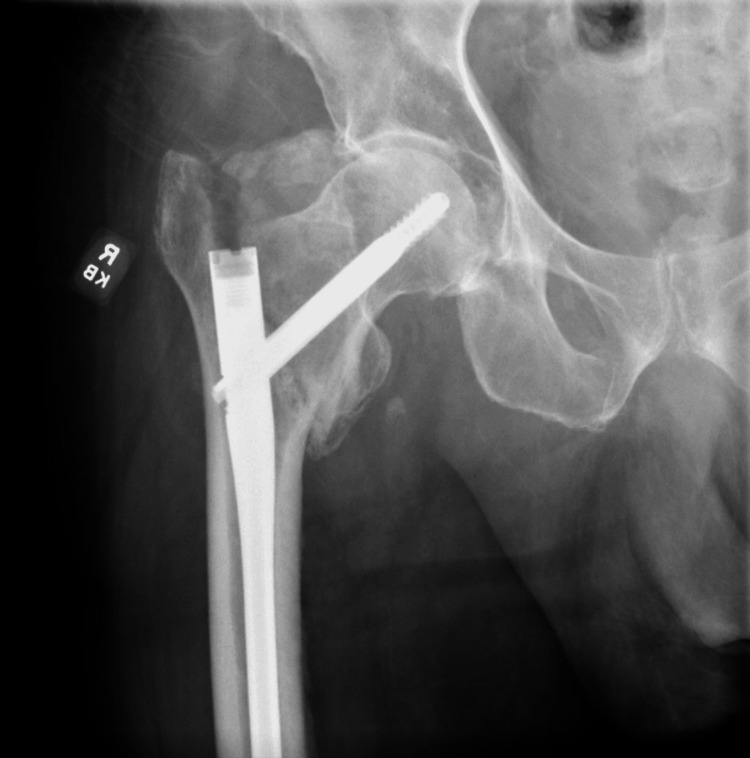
Preoperative anteroposterior radiograph of the right hip Anteroposterior view of the right hip showing a recurrent intertrochanteric fracture with inferior migration of the prior lag screw and subsidence of the intramedullary nail, consistent with implant failure.
